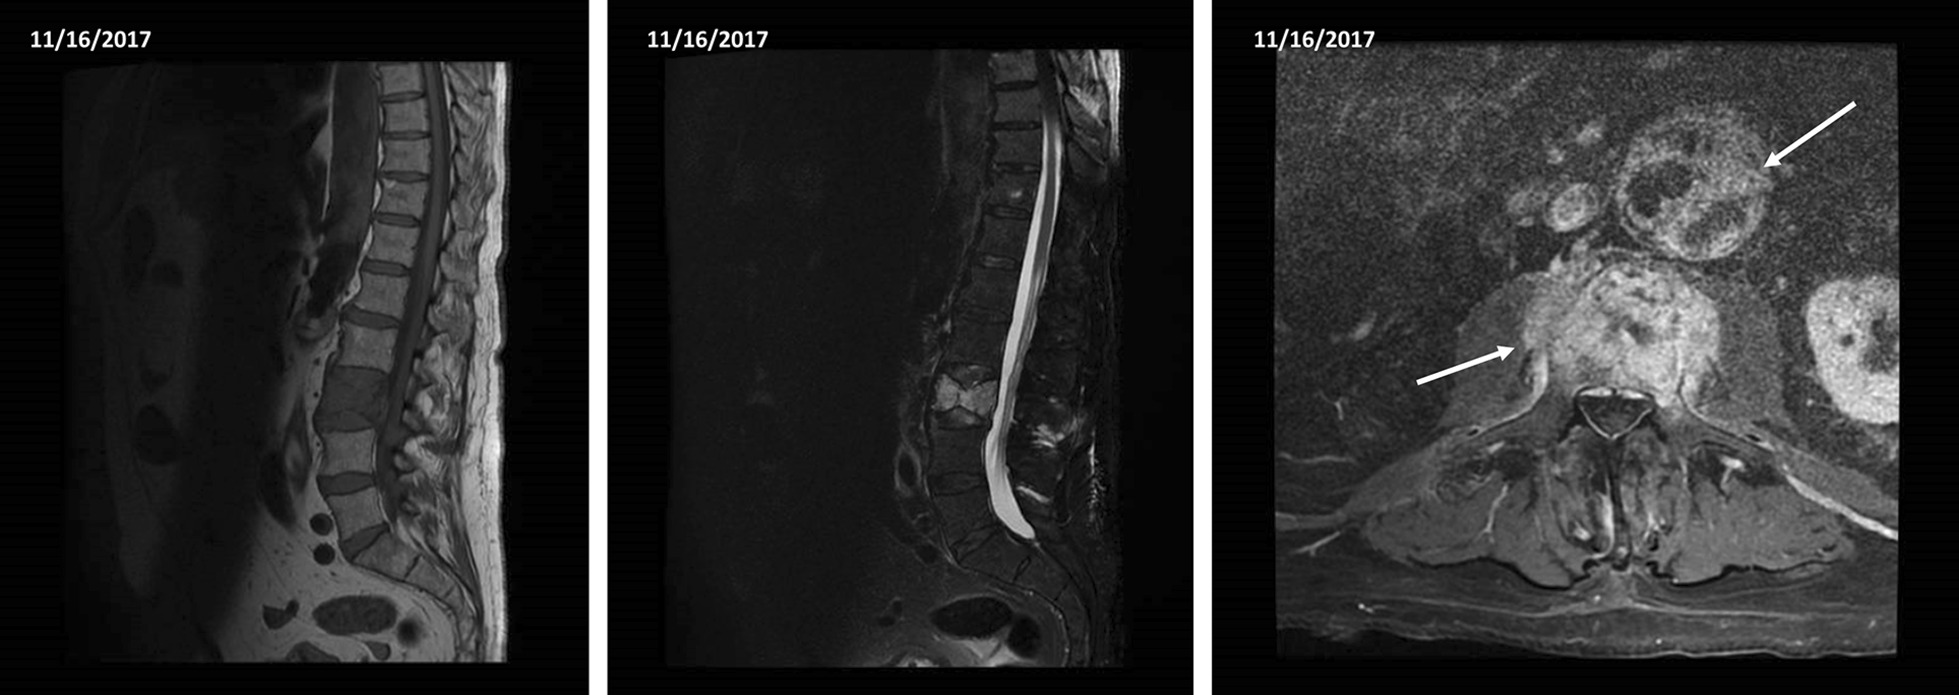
MRI imaging showing the vertebral alteration L3 and the inhomogeneous enhancement of the aortic sac
